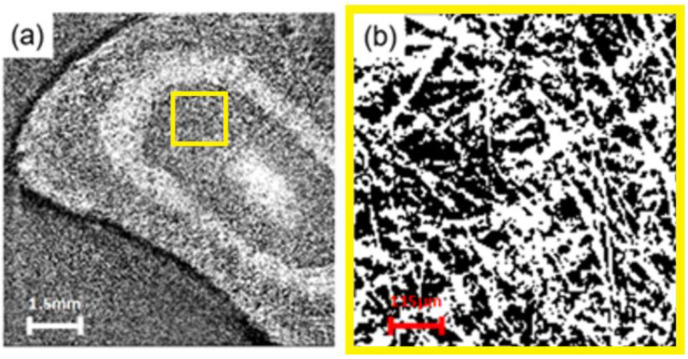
(a) Raw image of CT scans and (b) zoomed image of selected area. Adapted from [47].
